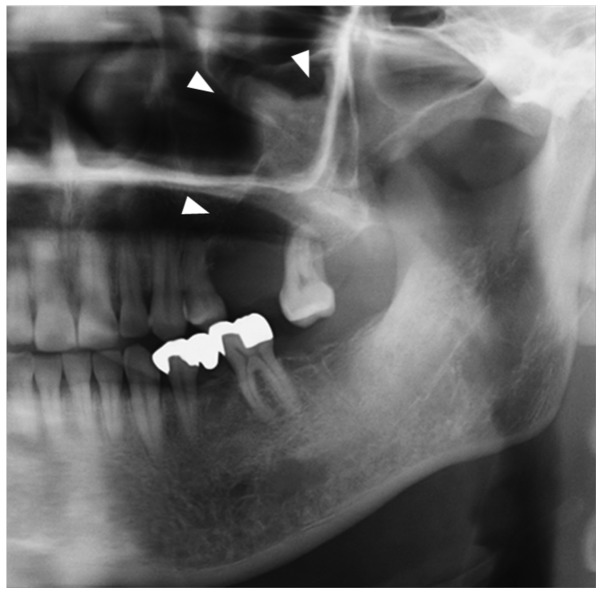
A panoramic radiograph revealed a dome-shaped radiopaque mass with well-defined margins extending from the left maxilla to the maxillary sinus.
